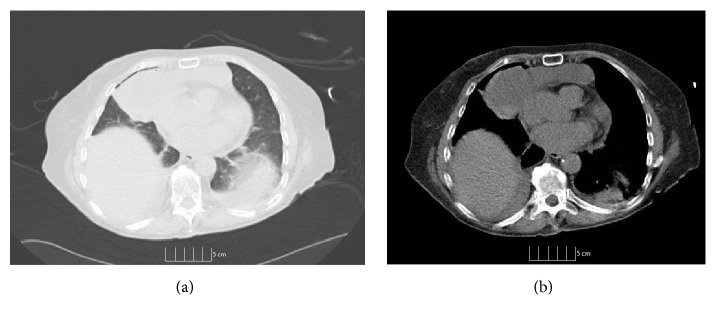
Cystic lesion in the mediastinum on (a) noncontrast CT lung window and (b) noncontrast CT of the chest.
